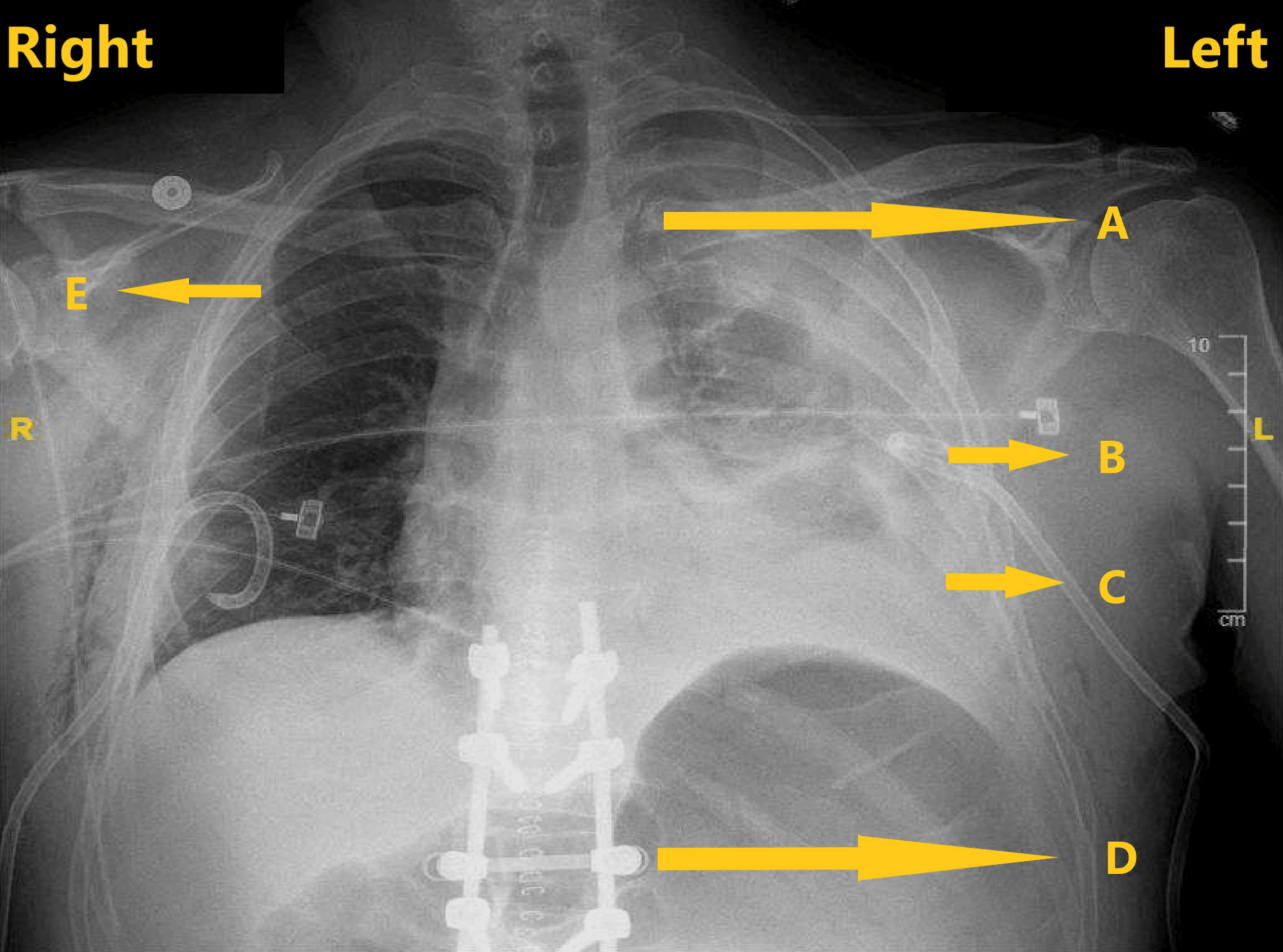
Chest radiography demonstrating rib fractures, pneumothorax, hemothorax, chest tubes and postsurgical hardware in spine A) rib fracture; B) chest tube; C) hemothorax; D) postsurgical hardware; E) pneumothorax
